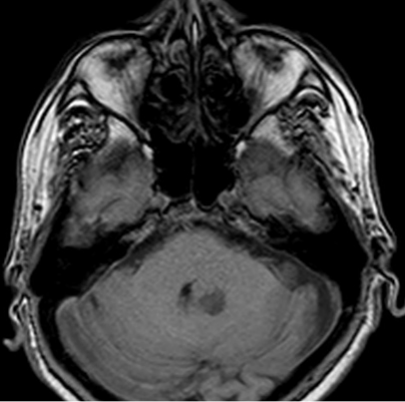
Axial T1 weighted magnetic resonance image of 4th ventricle choroid plexus carcinoma
